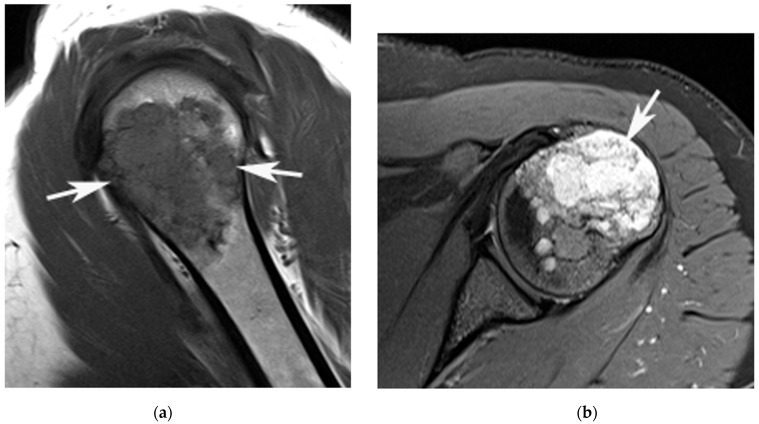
A 33-year-old female with an ACT in the left proximal humerus. (a) Sagittal T1W TSE and (b) axial SPAIR MR images show a chondral tumour (arrows) centred on the proximal metaphysis of the humerus. The lesion results in mild expansion of the lateral humeral cortex (arrow (b)) with no associated cortical breach.
